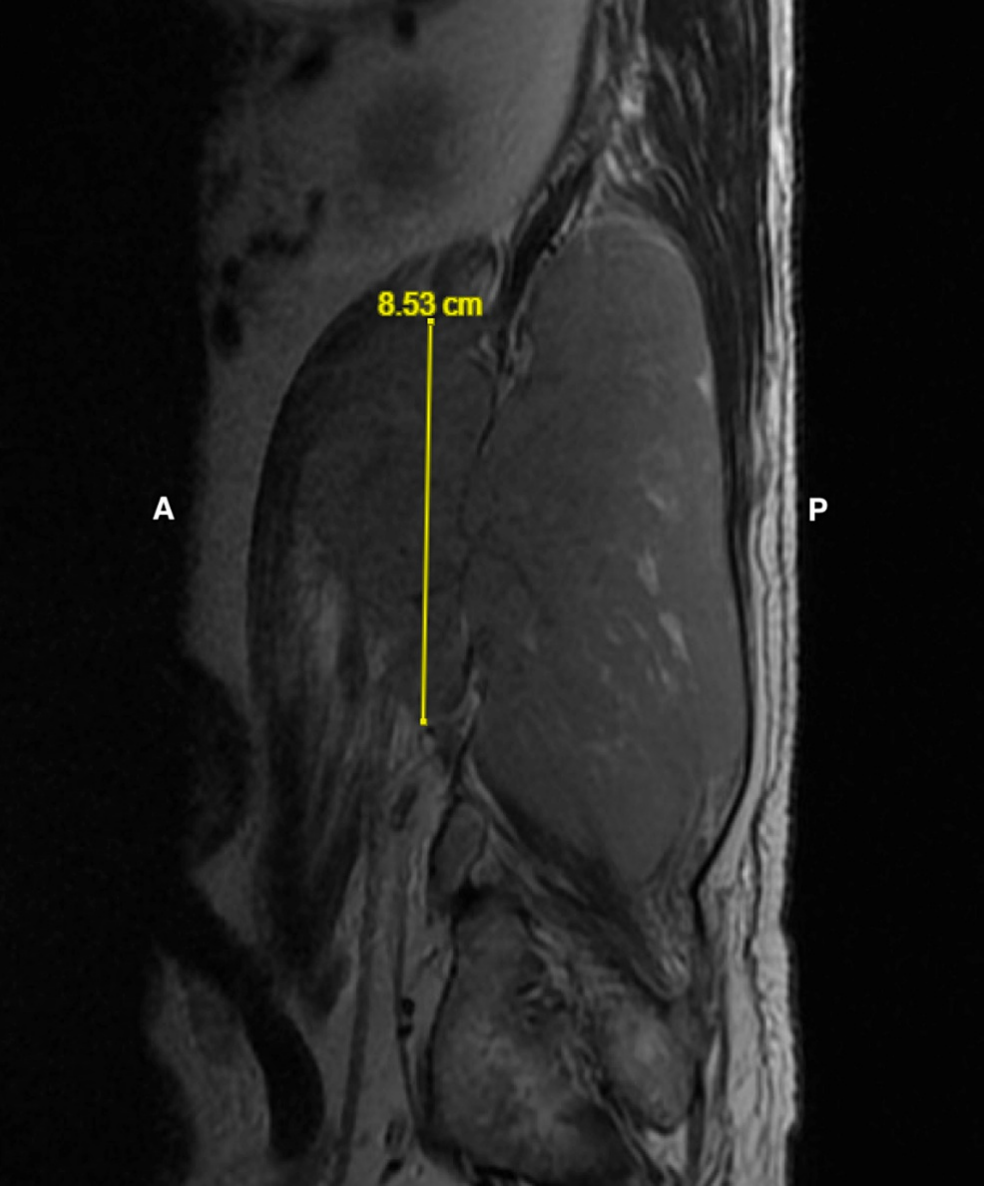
Anteriorly positioned paraspinous element extends roughly 8.53 cm craniocaudally in T2-weighted sagittal view A = Anterior; P = Posterior
